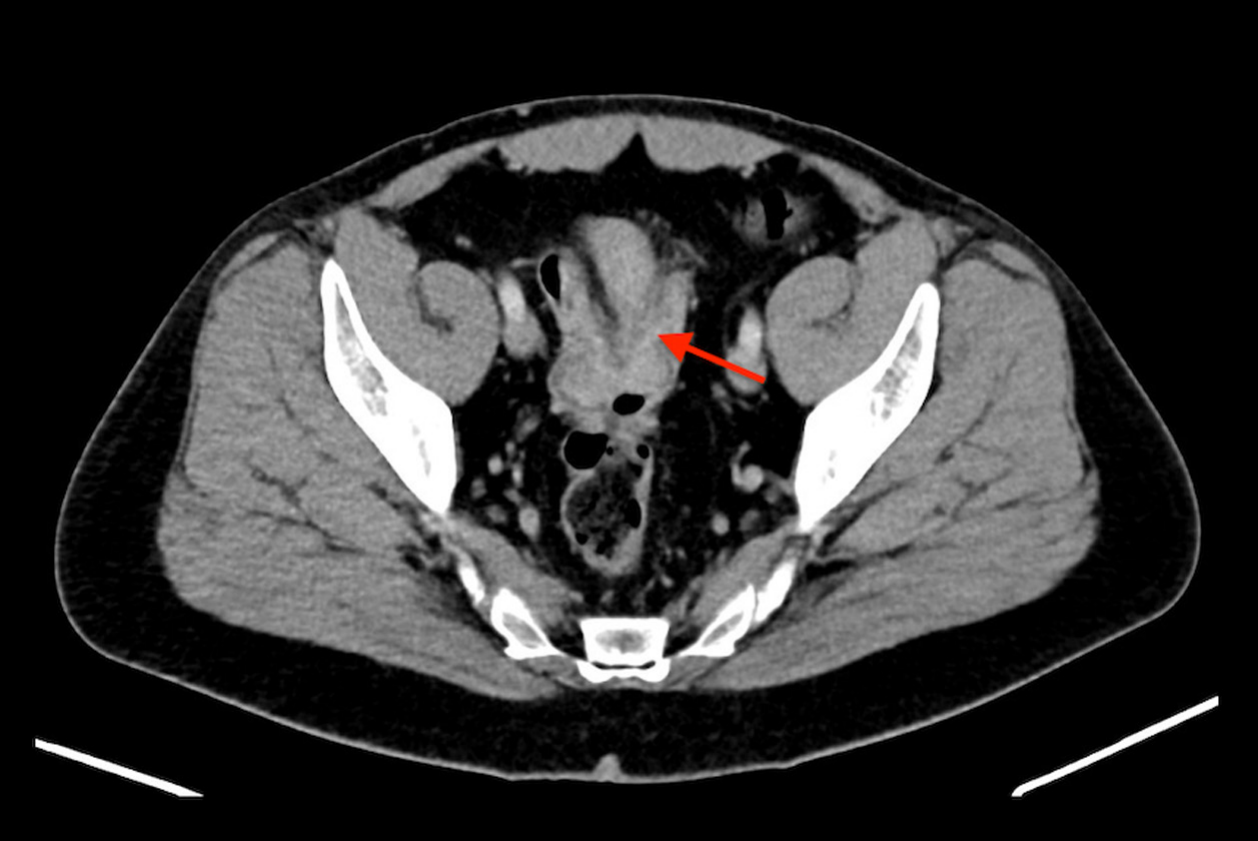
Abdominal computed tomography of the patient showing a tumor mass of the sigmoid colon.
